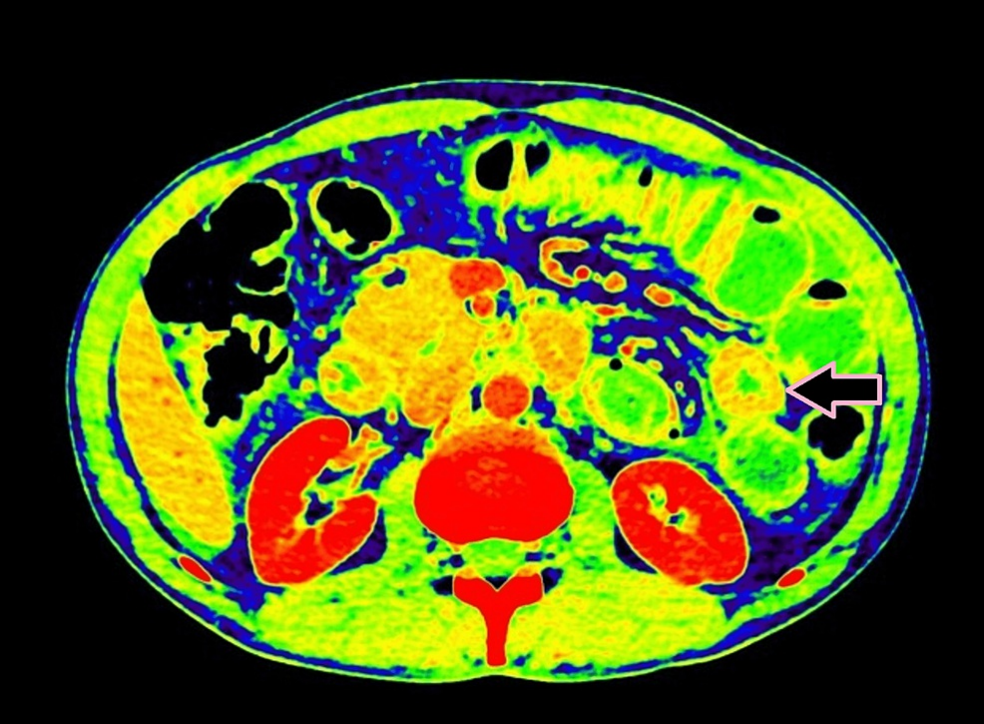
Axial DECT image on the basic atomic number of different metabolites present within the cells showing circumferential mural thickening of jejunum with luminal narrowing (case 1). DECT: dual-energy computed tomography
